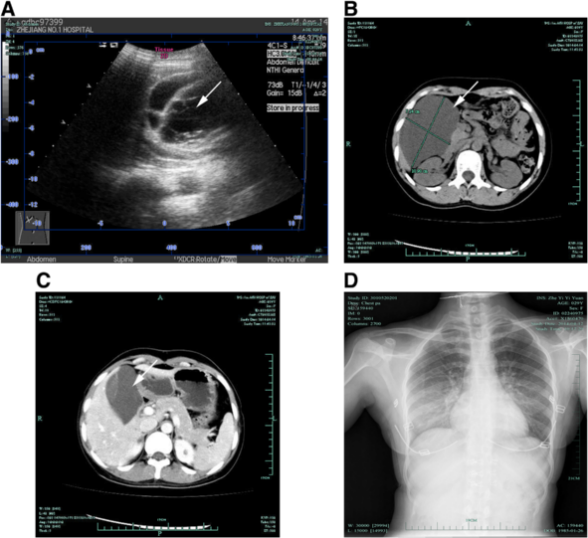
Image findings. Abdominal ultrasonography (US) showed a right abdominal cystic mass with segmentation, and the presence of a lymphatic cyst was considered (A). An abdominal contrast-enhanced CT scan revealed a large cyst between the liver and stomach, approximately 10.47 × 66.10 cm in size (B). The density of the cyst was homogeneous, and no obvious contrast signal was noted (C). Chest X-ray showed no significant abnormalities before the operation (D).
